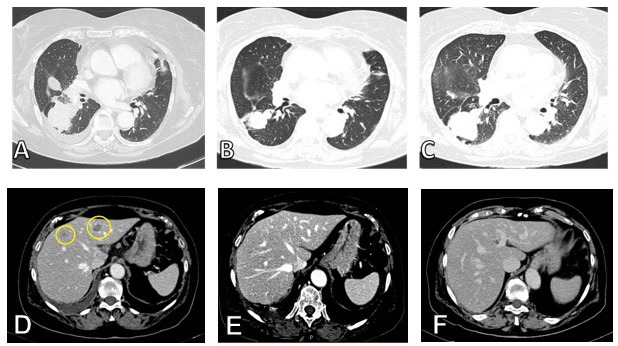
A. Thoracic CT scan of primary lesion before treatment with CBCDA + VP -16, B. Response after 3 cycles of CBCDA + VP -16 and C. progression after 5 cycles of CBCDA + VP -16. D. Liver metastases before treatment with CBCDA + VP -16, E. complete response after 3 cycles and F. persistence of complete response after 5 cycles of CBCDA + VP -16.
